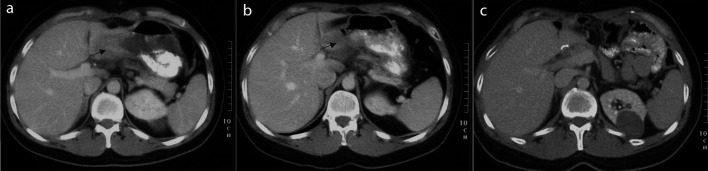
Abdominal CT scans. a) before treatment December 2003
